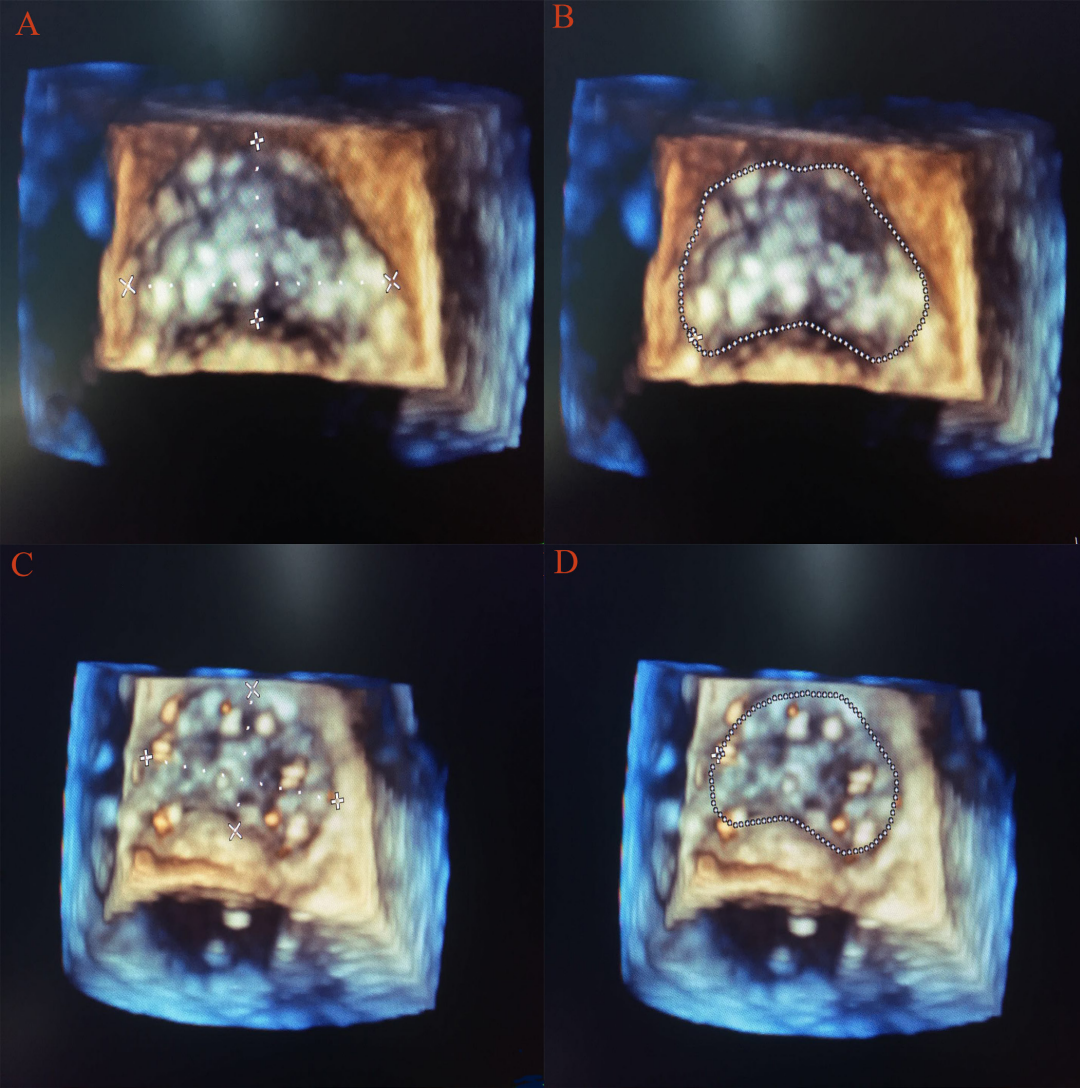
Changes in 3D mitral valve model after aortic valve replacement (AVR). Screen capture demonstrates a completed 3D mitral valve model. The 2D long-axis echocardiographic views represent orthogonal planes with a cross-sectional coronal plane for annular area tracing. The 3D model shows the final model with automatically labeled landmarks and diameters. A, B=Pre-AVR analysis. C, D=Post-AVR analysis in the same patient demonstrating a reduction in the mitral annular area (from 5.91 to 4.25 cm2), anterior-posterior diameter (from 1.92 to 1.68 cm), and anterolateral-posteromedial diameter (from 3.80 to 3.27 cm).
